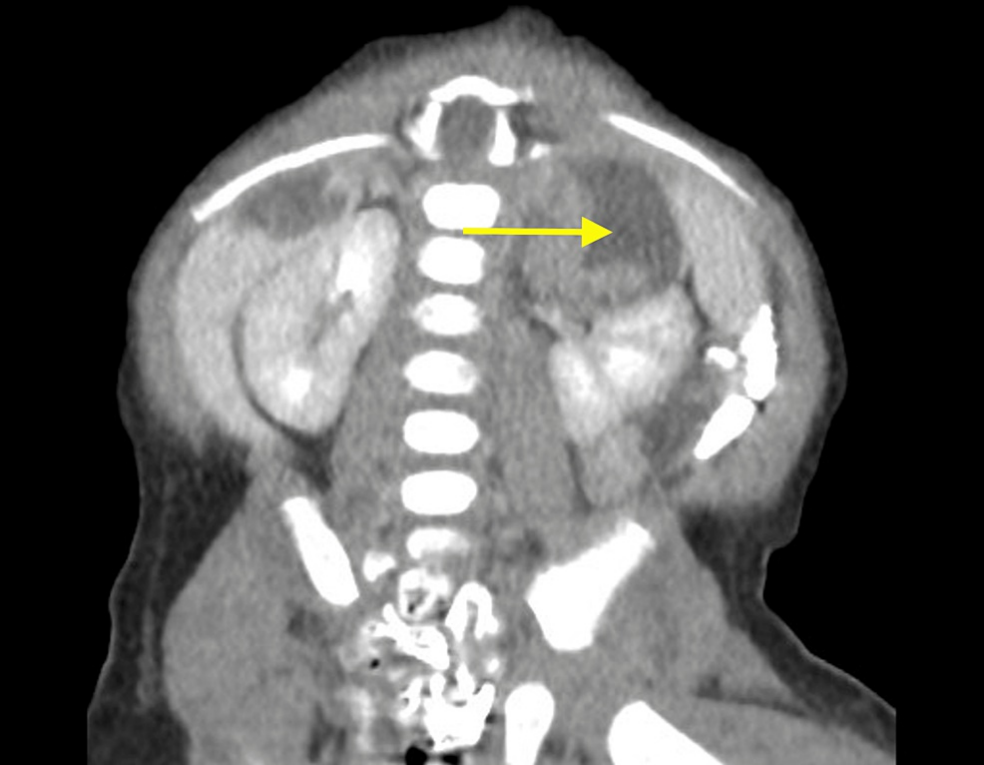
Coronal enhanced CT demonstrates well-circumscribed, fluid-attenuating cysts lateral/posterior margin of the liver There is a cyst seen in the left para-renal region, which appears to be contiguous with the left adrenal gland.
